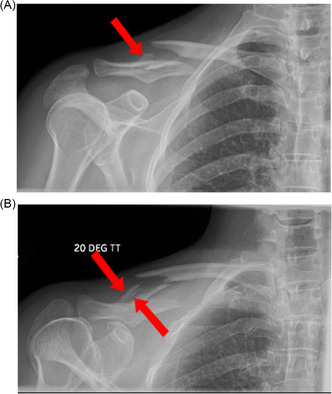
(A) Anteroposterior (AP) radiograph of the right clavicle with no tilt. (B) AP radiograph of the right clavicle with 20° of cephalad tilt. Red arrows are highlighting posterior butterfly fragments.
